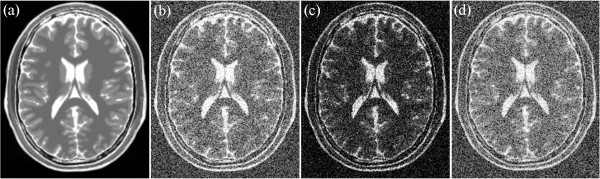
An example of T2 image: (a) original T2 weighted MRI, (b) 17% noisy T2 weighted MRI, (c) squared magnitude of the noisy T2 weighted MRI, (d) the forward variance-stabilization transformation of the noisy T1 weighted MRI.
